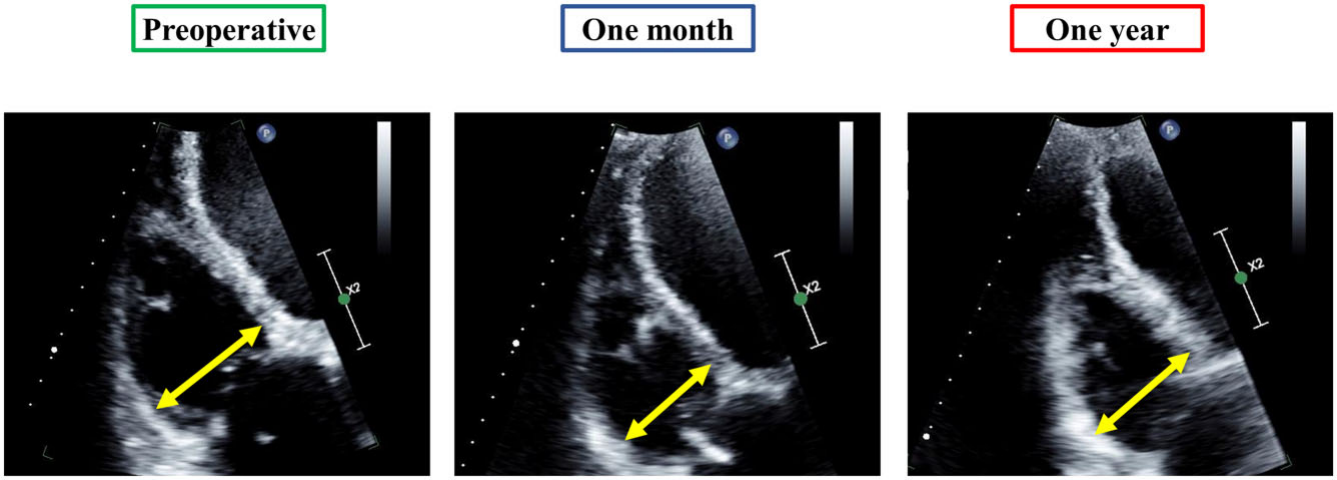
Changes in the RV geometry after LT approach in modified apical four-chamber views. RVEDD: preoperative, 50 mm; 1 month, 42 mm; 1 year, 47 mm. RVEDA: preoperative, 40 cm^2^; 1 month, 28 cm^2^; 1 year, 32 cm^2^. Yellow arrow: RVEDD. LT: lateral thoracotomy; RV: right ventricle; RVEDA: right ventricular end-diastolic area; RVEDD: right ventricular end-diastolic dimension.
